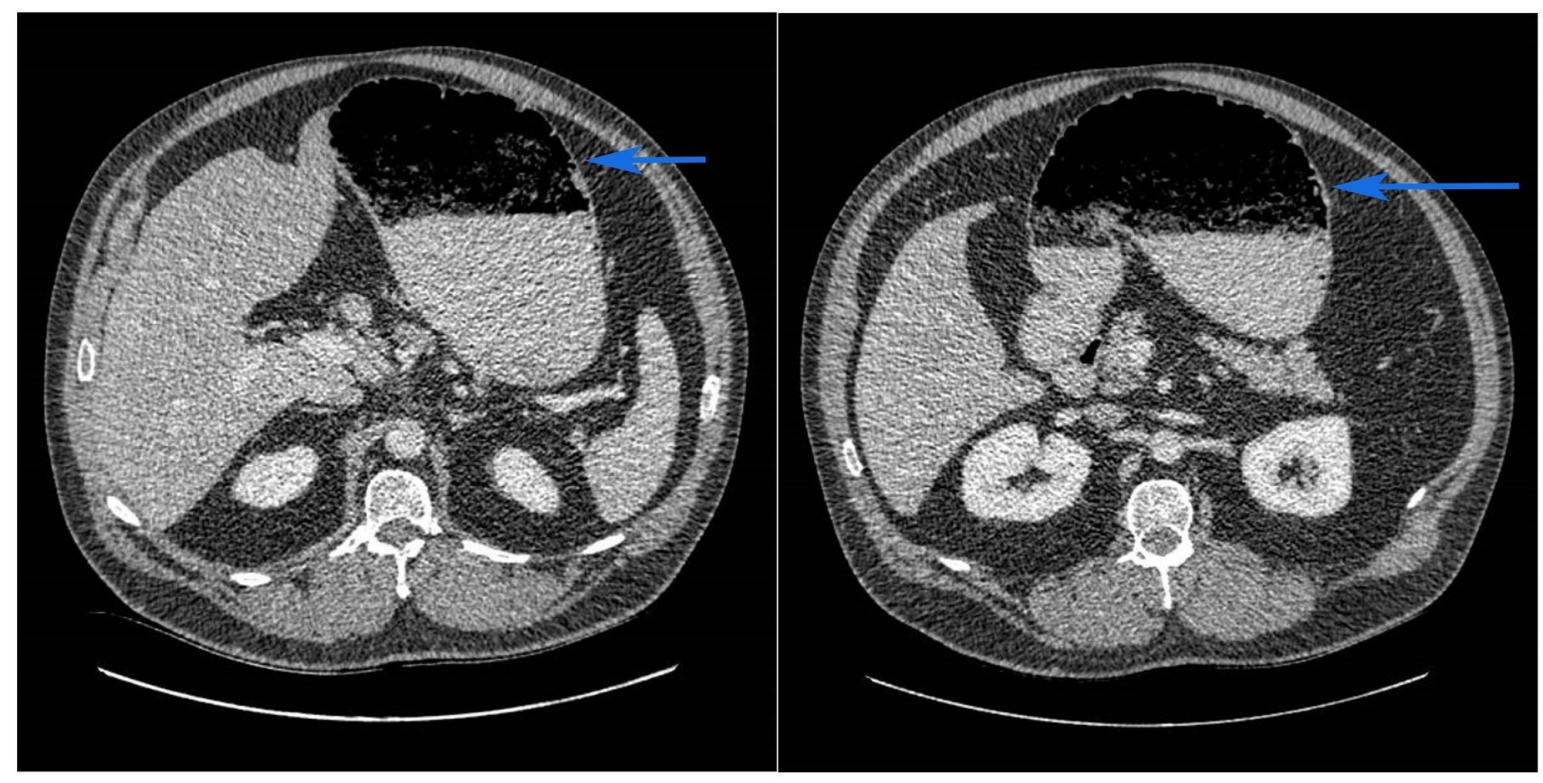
Computed tomography (CT) scan of the abdomen CT scan of the abdomen showing a markedly distended stomach (blue arrow) with food/debris and normal caliber duodenum without an obvious lesion
